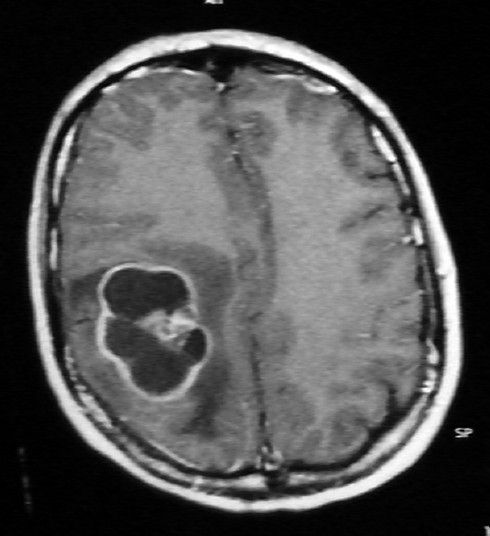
Cranial axial MRI with gadolinium revealing a ring-enhancing 4 × 4.5 cm mass lesion in the right parietal lobe with significant surrounding peripheral edema.
